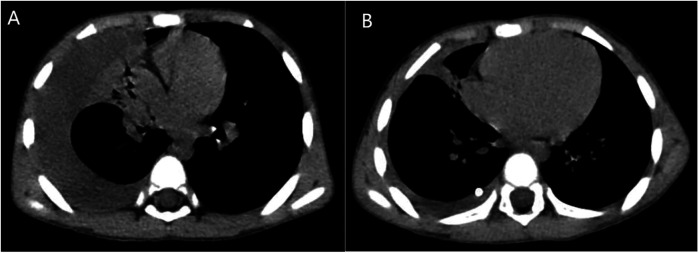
Chest CT (mediastinal windows) of the patient before (A) and after (B) treatment.
